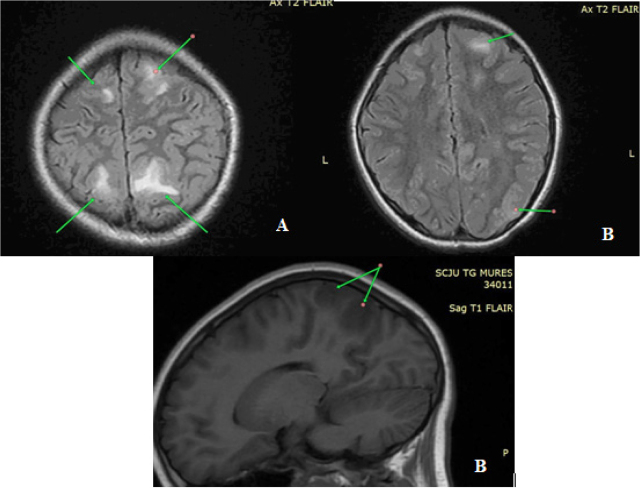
An inflammatory cerebral lesion showing hypersignal on T2 and FLAIR sequences, located at the level of the bilateral frontal lobe and post-central gyrus (A), respectively on the left parietal side (B)
